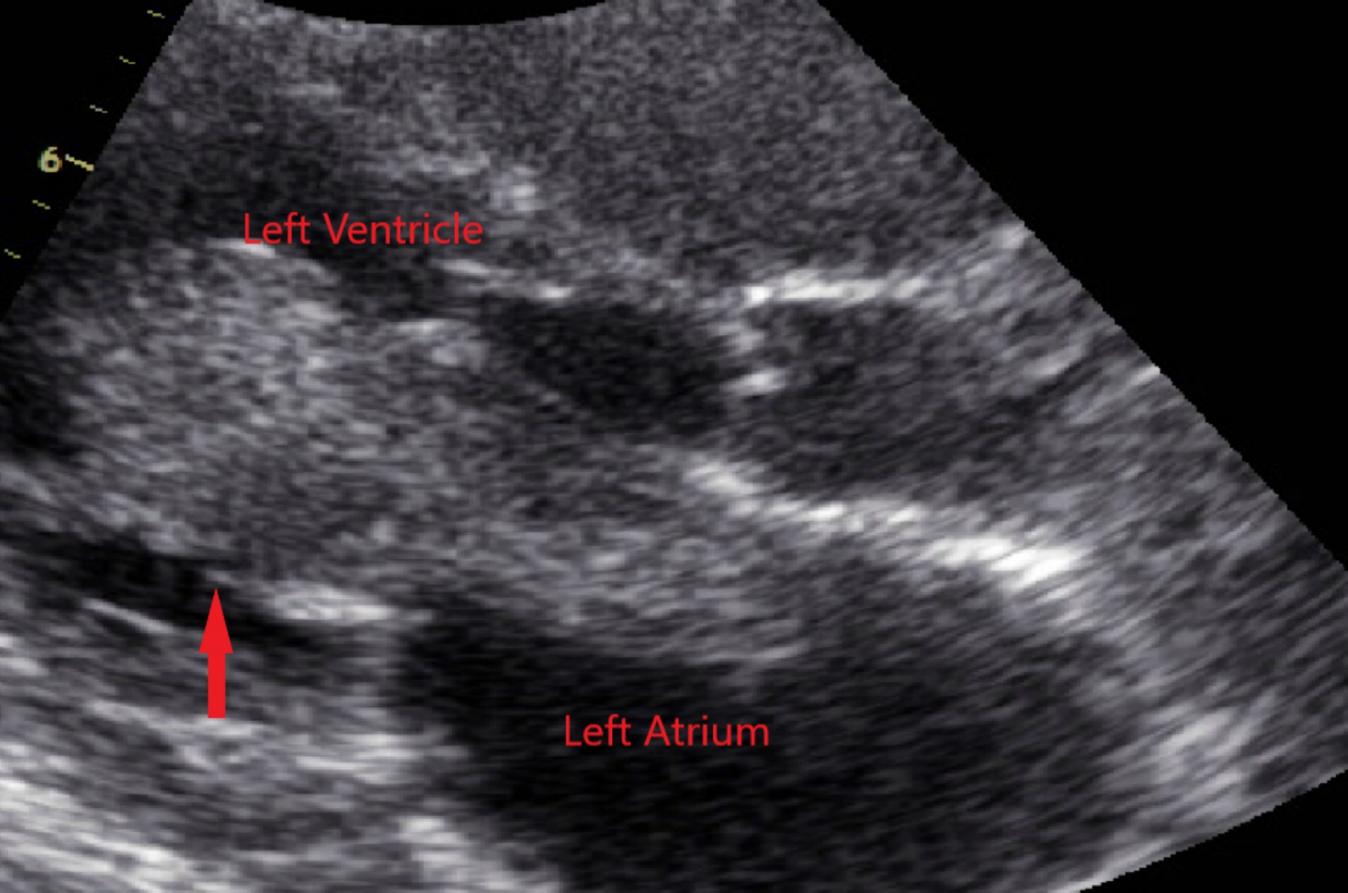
Parasternal long axis on transthoracic echocardiogram showing prolapse of the large left atrial mass into the left ventricle
